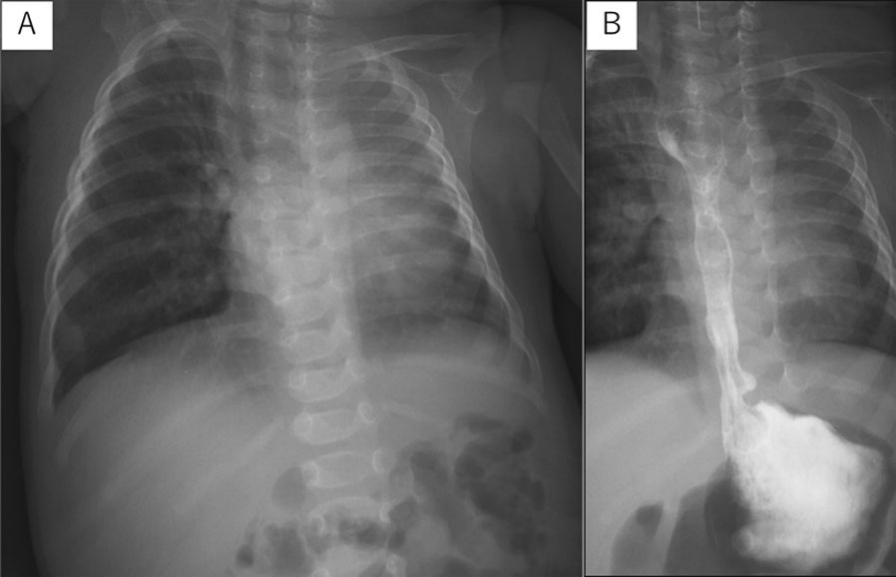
Postoperative chest radiography showing a well aerated left lung (A). Esophagography showing satisfactory passage at the anastomotic site (B)
